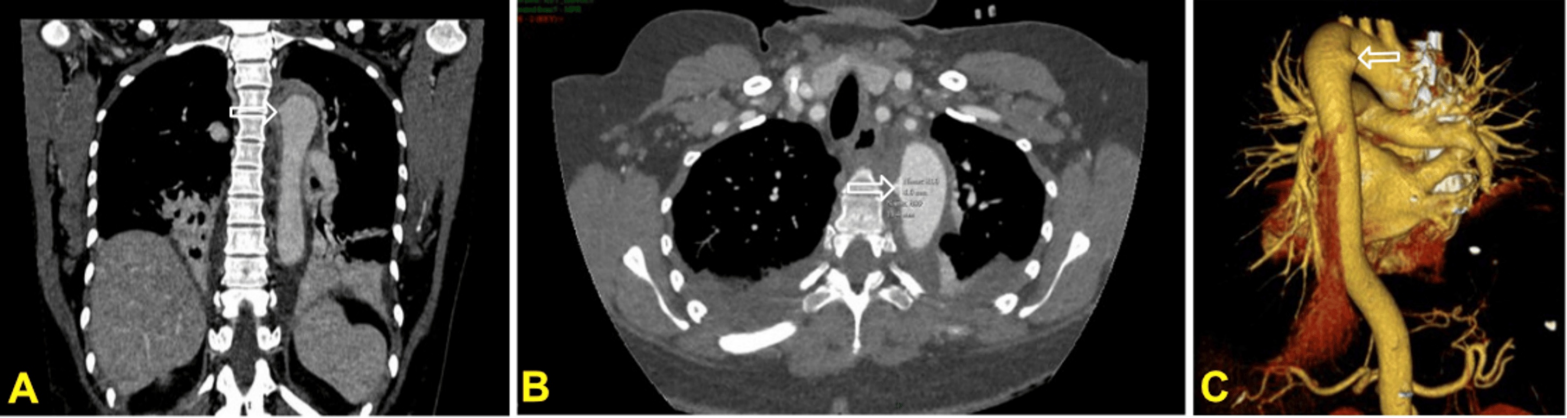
CT scan demonstrated a pseudoaneurysm (white arrows) at the previous location of the fish bone (at the level of T3 vertebra), measuring 4 mm × 10 mm CT: computed tomography; CTA: computed tomography angiography Mild bilateral pleural effusion was noted. (A) and (B), coronal and axial planes; (C), CTA volume-rendered reconstruction. The white arrow (A, B, and C panels) demonstrated the aortic pseudoaneurysm
